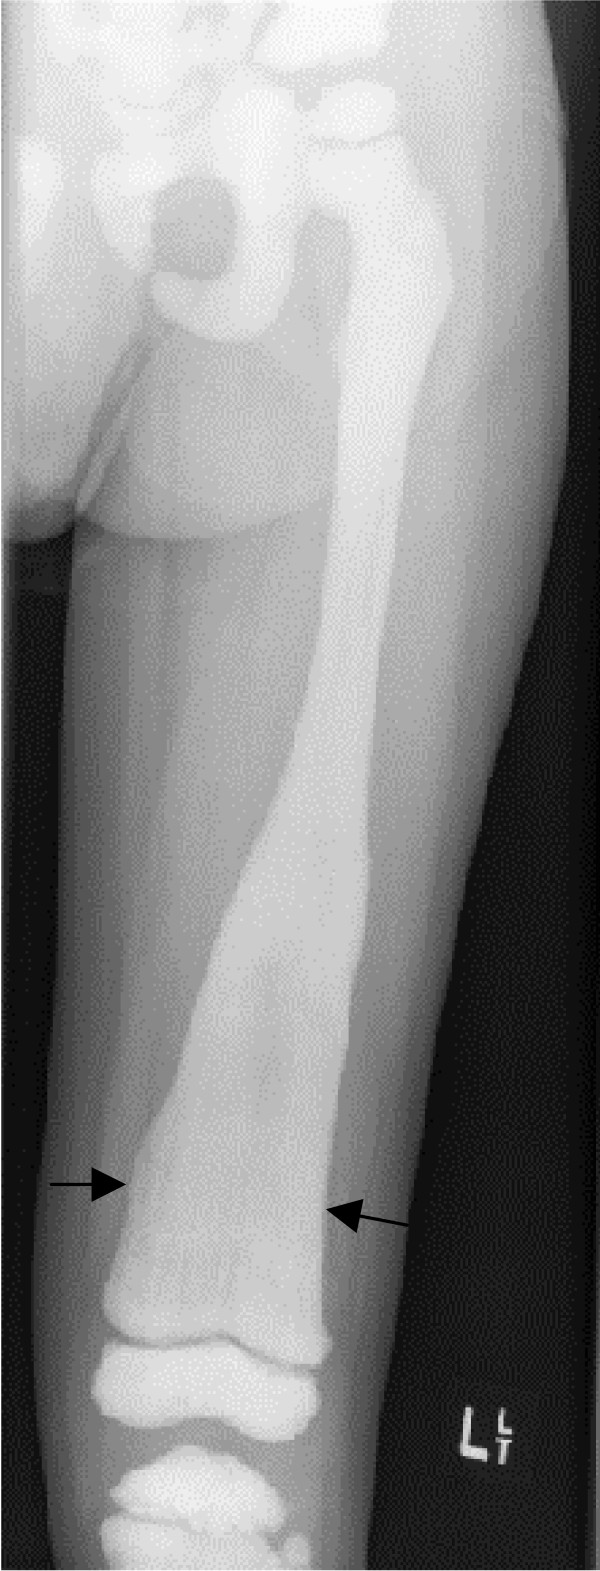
ADO: Radiograph of left femur, age 4 years. Note Erlenmeyer flask deformity of distal femur (arrows) and generalised increased bone density.
